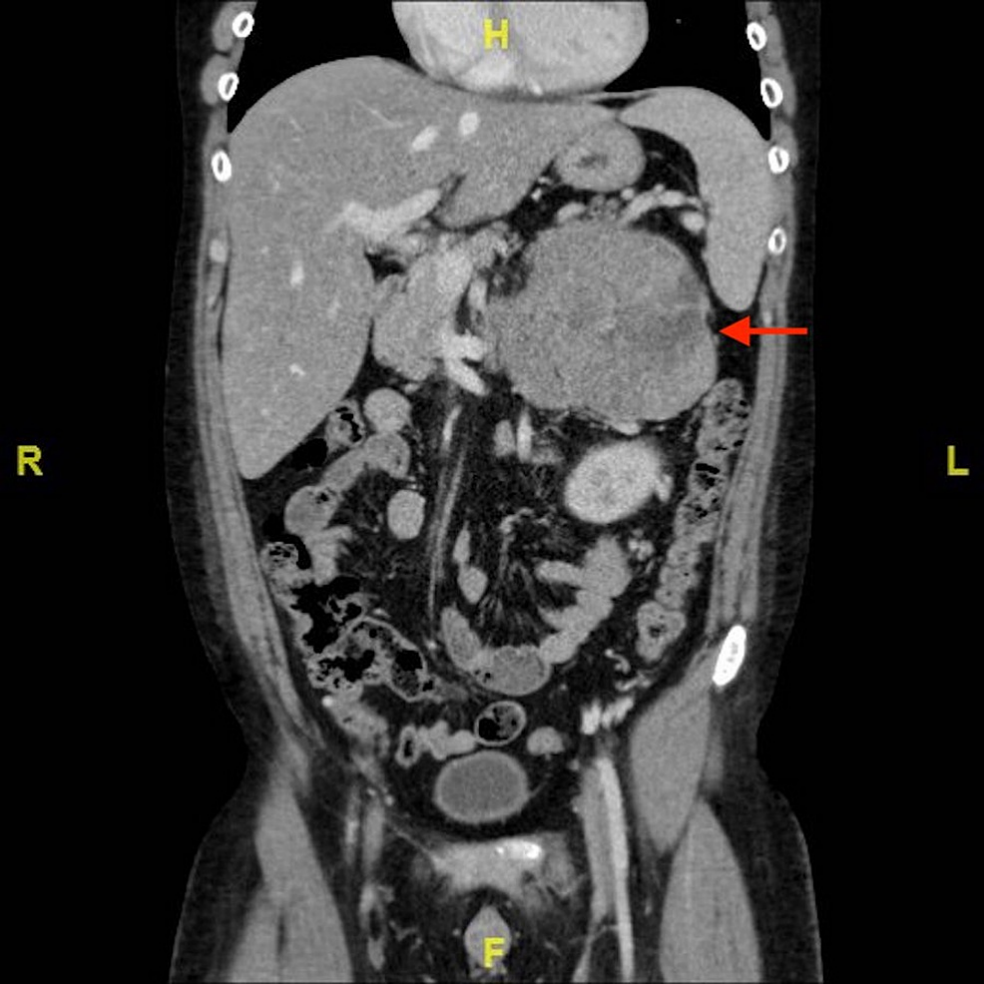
CT scan shows a large heterogeneous mass in the area of the left adrenal gland (red arrow), with numerous collateral vessels.
